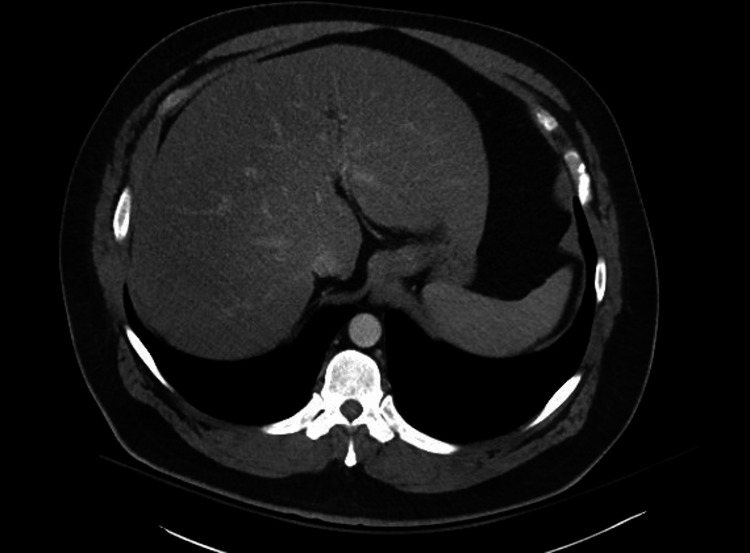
Computed tomography (CT) abdomen/pelvis showing fatty liver with no obstruction, masses, or stones.
